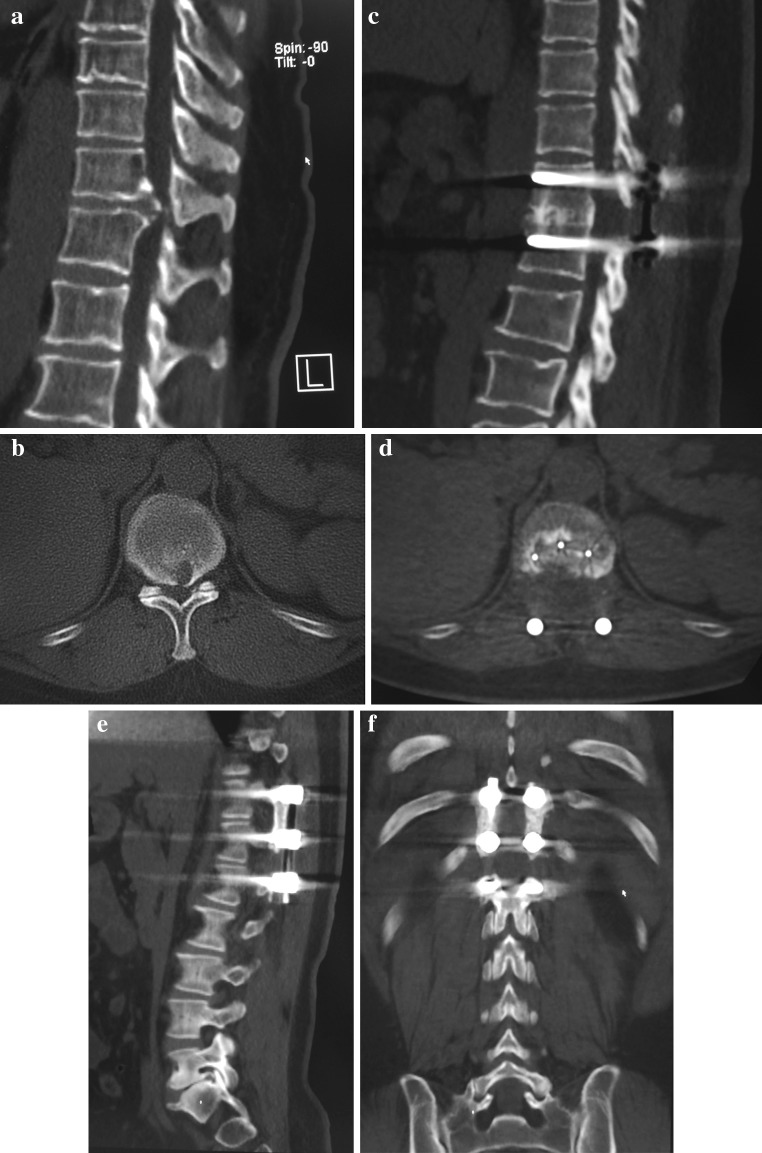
Pre-operative CT images (a, b) show the ventrally placed hard disc. Post-operative CT images (c, d) show that the herniated disc had been completely excised and the interbody fusion was solid. Images (e, f) demonstrate solid posterolateral fusion in another patient
